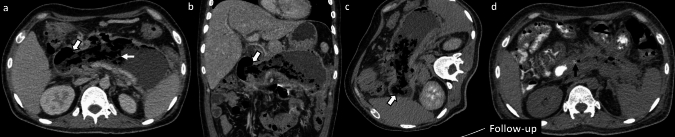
Air within necrotizing pancreatitis due to spontaneous enteric fistula. A 40-year-old with acute necrotizing pancreatitis. Initial CT images a–c show large WON replacing the entire pancreas with multiple air foci (solid white arrow, a). The WON is communicating with the duodenum (white block arrows, a, b). With right lateral decubitus (c), air and fluid gravitate into the second portion of the duodenum (white block arrow, c). Follow-up CT with enteric contrast d shows nearly resolved WON. The positive duodenal enteric contrast leaks into the pancreatic bed (black arrow, d)
